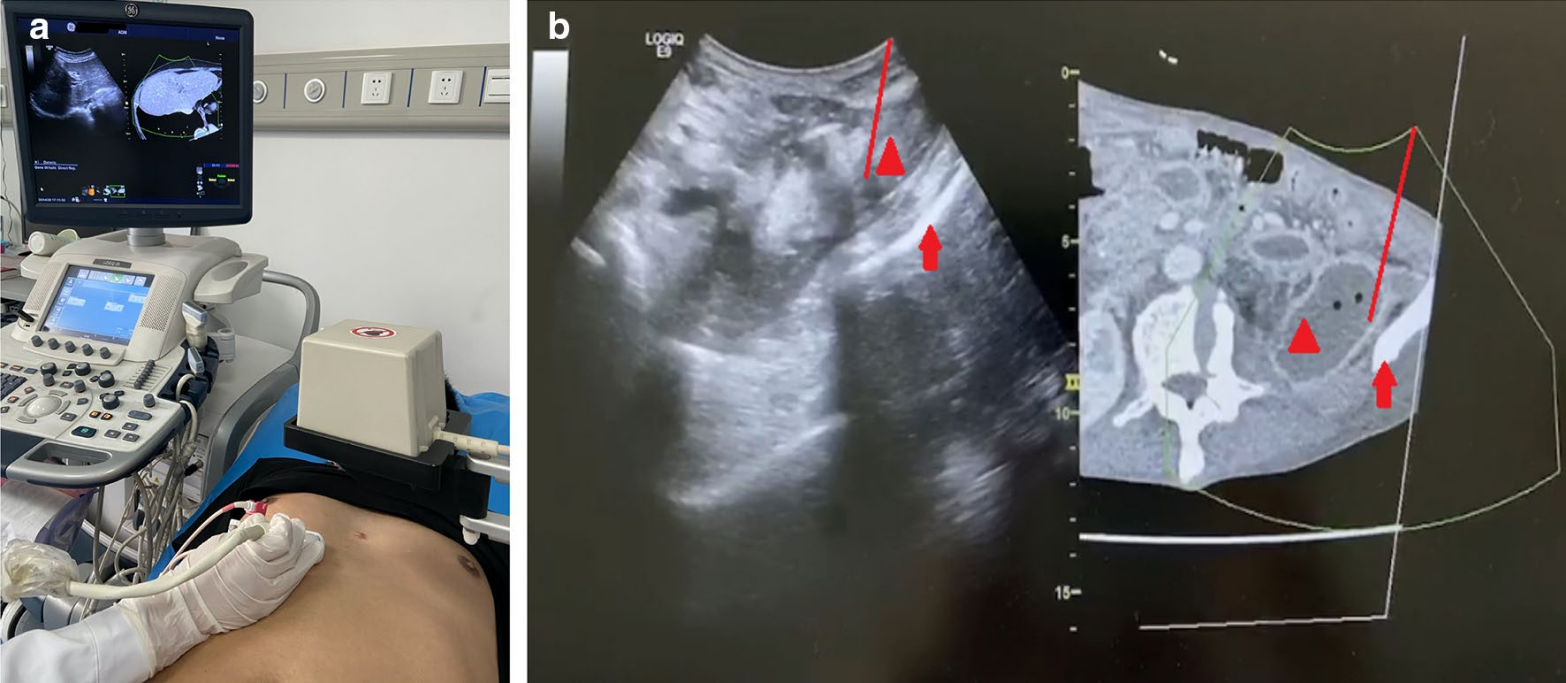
Selection of the puncture pathway under the guidance of an ultrasonography (US)/computer tomography (CT) imaging fusion system. a The US/CT imaging fusion system consists of a magnetic sensor-loaded ultrasonic detector, magnetic generator, and magnetic receiver, and the images of the liver are used for initial calibration. b The integrated image is displayed on the screen, puncture pathway (left midaxillary line) is indicated by red lines, walled-off necrosis (retroperitoneal area behind the colon) is indicated by red triangles, and the left ilium is indicated by red arrows
